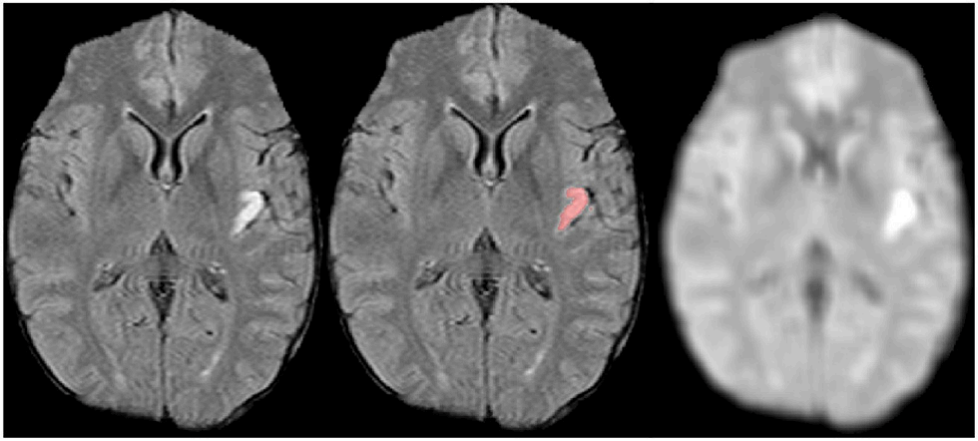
FLAIR MRI dataset (Left) with corresponding lesion segmentation (Center) and dominant Gabor texture coefficient image (Right). Since the lesion is rather small, the middle (second octave) component Gabor function at 45° orientation to the x-axis dominates.
